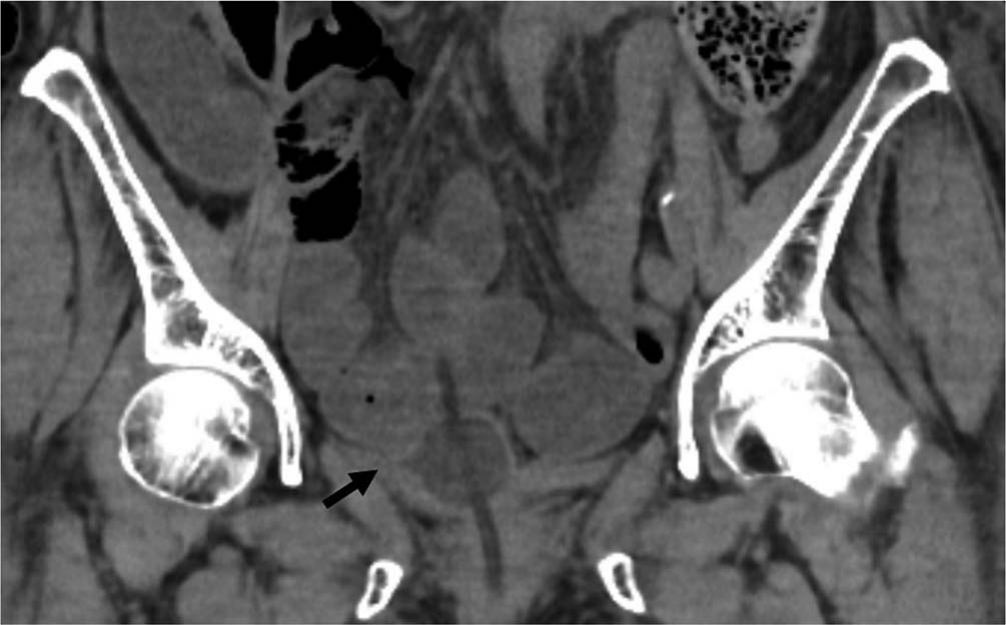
Coronal CT showed the urinary catheter was mispositioned outside the bladder (arrow).
